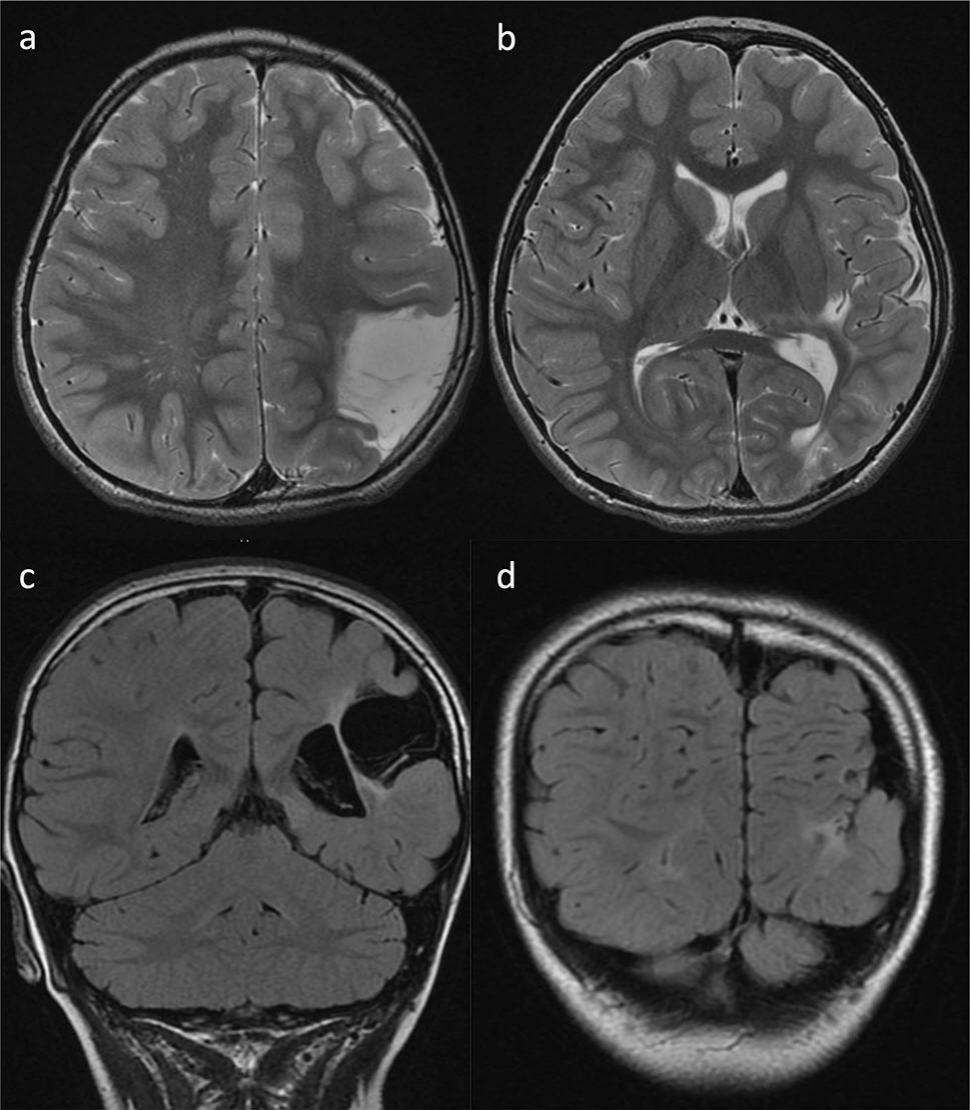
COL4A2*-related disorder in a 5-year-old, male patient with right hemiparesis. Axial T2-weighted imaging (a, b) and coronal FLAIR imaging (c, d) demonstrated cystic encephalomalacia in the left parietal lobe, ulegyria in the left insula and occipital lobe, and hyperintensities in the left posterior limb of the internal capsule and thalamus
